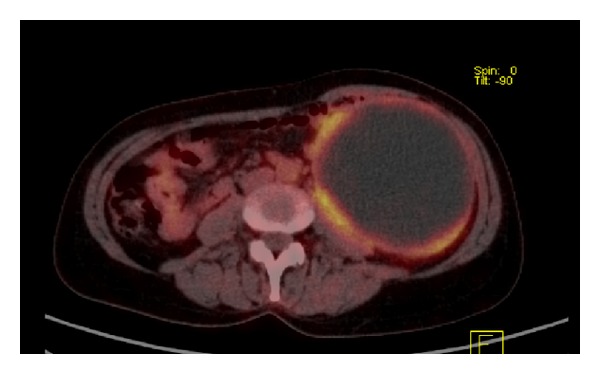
PET/CT scan of the abdomen (sagittal view) showing a cystic mass with peripheral uptake of 18-FDG.
